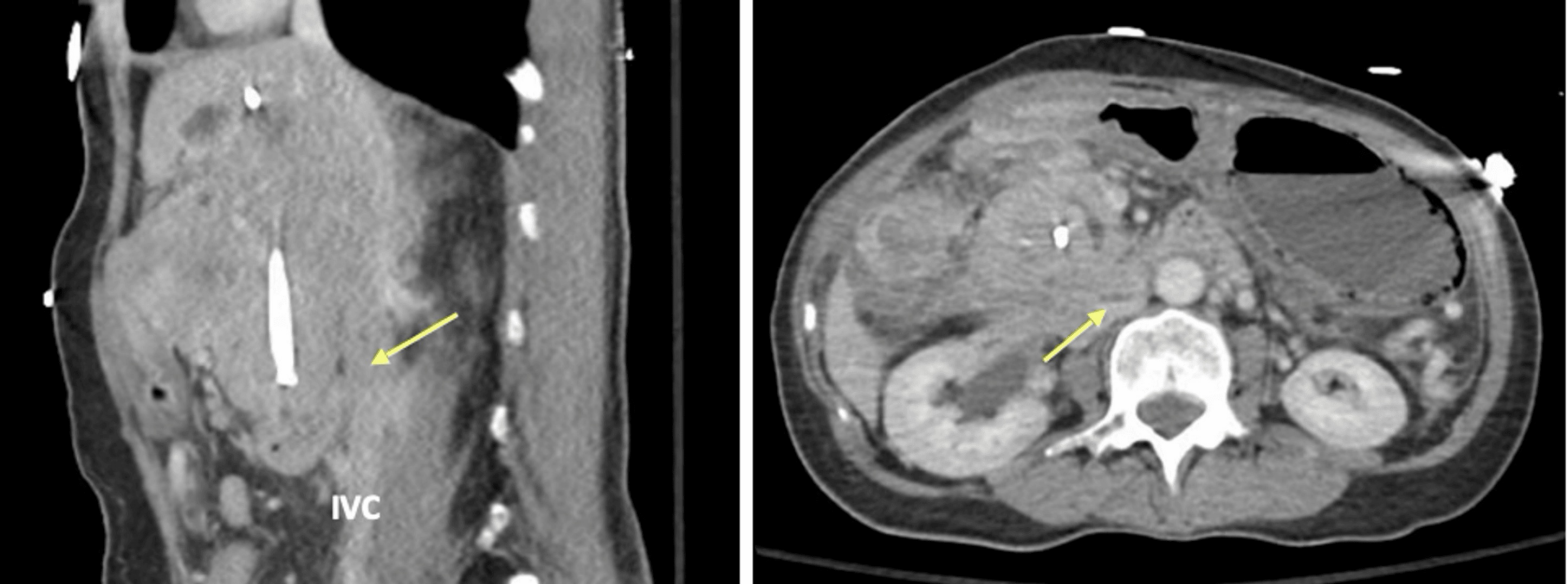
Sagittal (A) and Axial (B) views of CT AP demonstrating IVC invasion (yellow arrows) from the rapidly expanding gallbladder small cell carcinoma IVC: Inferior Vena Cava
